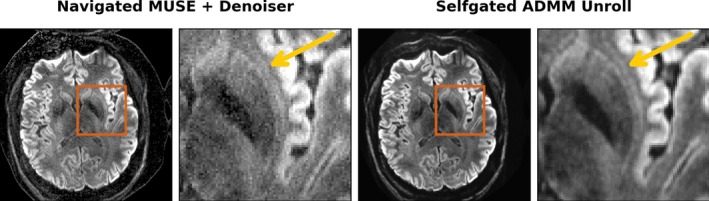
0.7 mm isotropic resolution DWI with the proposed self‐gated ADMM unrolling enables the visualization of the tiny structure claustrum, whereas the MUSE reconstruction shows only a blurred appearance. Displayed images are the mean diffusion‐weighted image from 20 directions and its zoomed‐in region.
